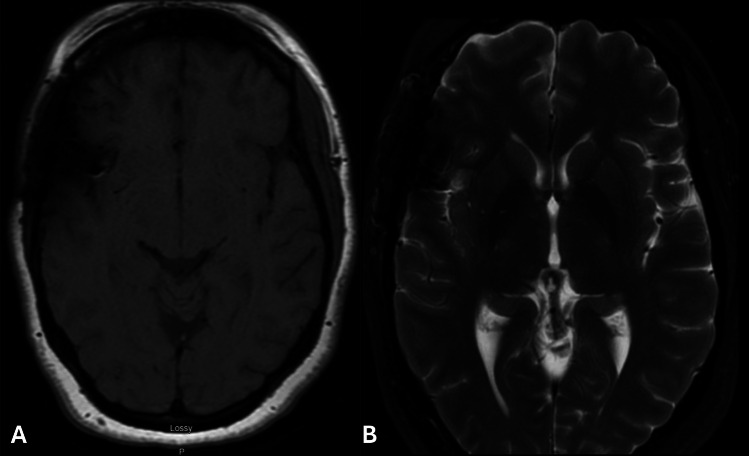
Post-operative brain imaging obtained two months after surgery demonstrates complete resection of the hyperintense lesion on axial T1-weighted MRI (A), while axial T2- weighted MRI (B) shows resolution of the perilesional edema
